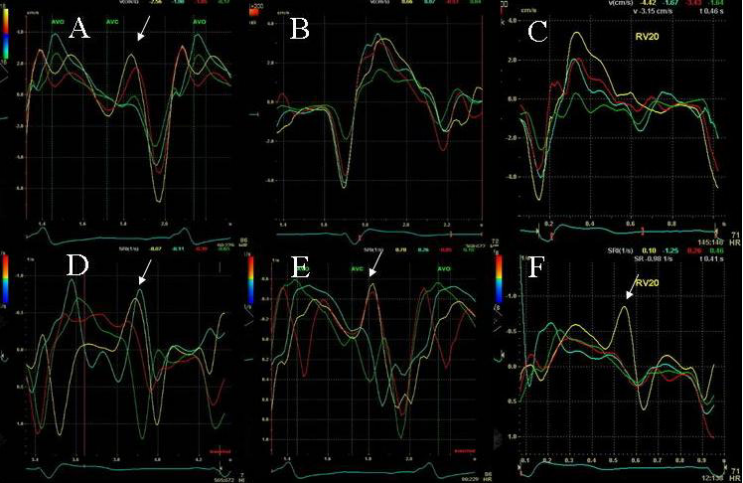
Tissue velocity (A, B and C) and strain rate (D, E and F) maps in the apical 2 chamber view at baseline pre cardiac resynchronization treatment (A and D), post cardiac resynchronization treatment with LV pre-excitation of 20 ms (B and E) and post optimization with RV pre-excitation of 20 ms (C and F). Note delayed longitudinal contraction (shown by white arrows) in the velocity (A) and strain rate maps (D) at baseline indicated by white arrows that is abolished in all segments in the velocity map in B and in mid inferior segment in the strain rate map in E post cardiac resynchronization treatment. In addition note the marked improvement in synchrony in systole between basal and mid segments post cardiac resynchronization treatment in B and E compared to A and D. RV pre-excitation causes further increase in velocities with establishment of a normal velocity gradient between basal and mid segments and improved synchrony in C, as well as improvement in strain rate velocities, synchrony and a reduction of delayed longitudinal contraction velocities in F. Note different velocity calibration settings between D-F. Yellow line represent basal inferior LV segment, blue represents basal anterior LV segment, red represents mid inferior LV segment and green mid anterior LV segment.
